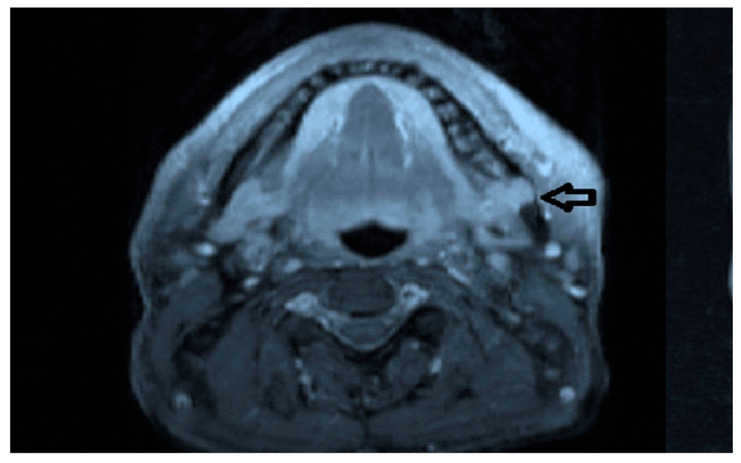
MRI view of the left retromolar region showing with the indication of black arrow a soft-tissue lesion corresponding to the clinical site of the Kaposiform hemangioendothelioma.
